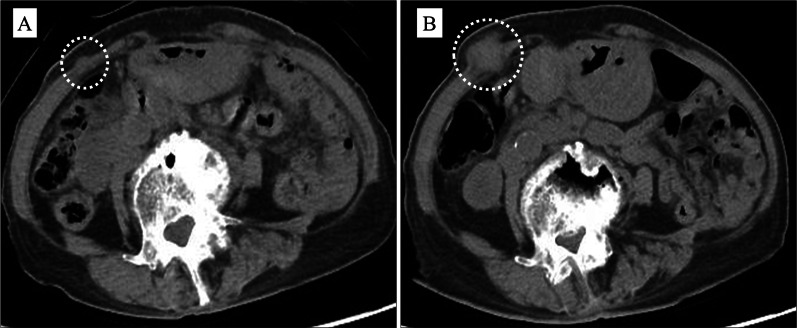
Computed tomography findings after distal pancreatectomy. A Five months after surgery, an abdominal wall tumor was found in the 5-mm port site in the right hypochondrium. B One year after surgery, the tumor had grown to a diameter of 25 mm. No other intraperitoneal recurrence was detected
